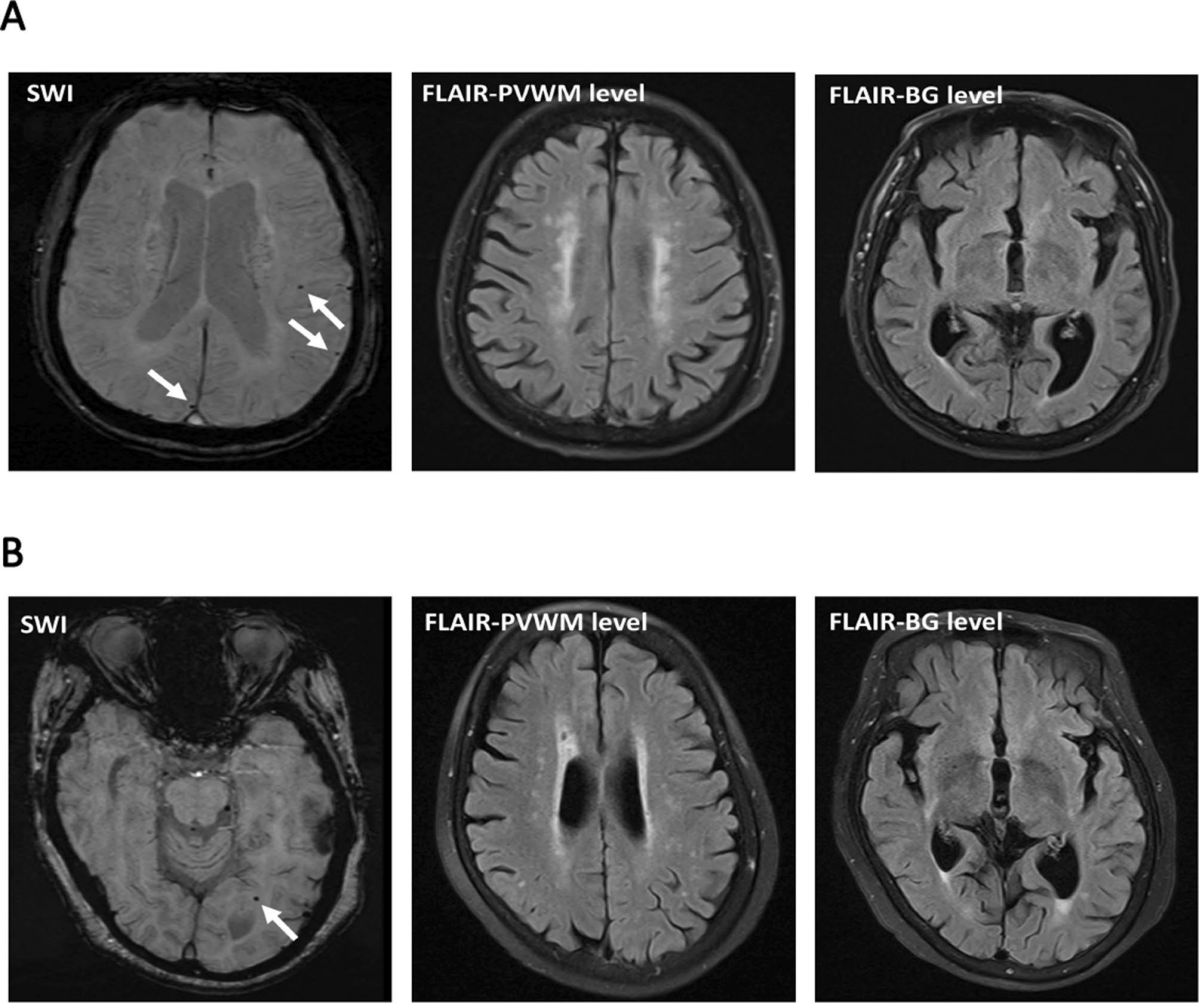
Representative images of lobar microbleeds in Parkinson’s disease. (A) A 66-year-old woman with Parkinson’s disease dementia (PDD, MMSE score 23) had multiple lobar microbleeds in the left parietal and right occipital lobes (arrow) and minimal white matter hyperintensity (WMH) on fluid-attenuated inversion recovery imaging. There was no lacunar infarct over bilateral BG. (B) An 82-year-old woman with PDD (MMSE score 16) had one lobar microbleeds in the left occipital (arrow), the left parietal, and the right temporal lobes (not shown). Mild WMH was also seen on fluid-attenuated inversion recovery imaging. There was no lacunar infarct over bilateral BG. *SWI* susceptibility weighted imaging, *FLAIR* fluid-attenuated inversion recovery imaging, *PVMW* periventricular white matter, *BG* basal ganglia.
